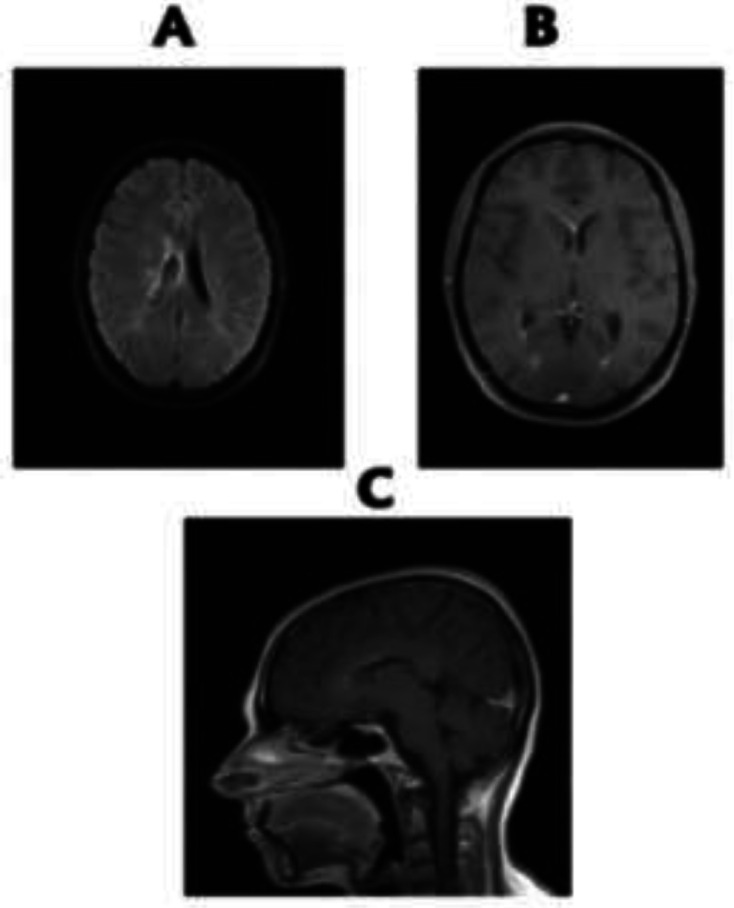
A. Brain MRI revealed periventricular lesions, B. periependymal enhancement and, C. linear enhancement of corpus callosum
